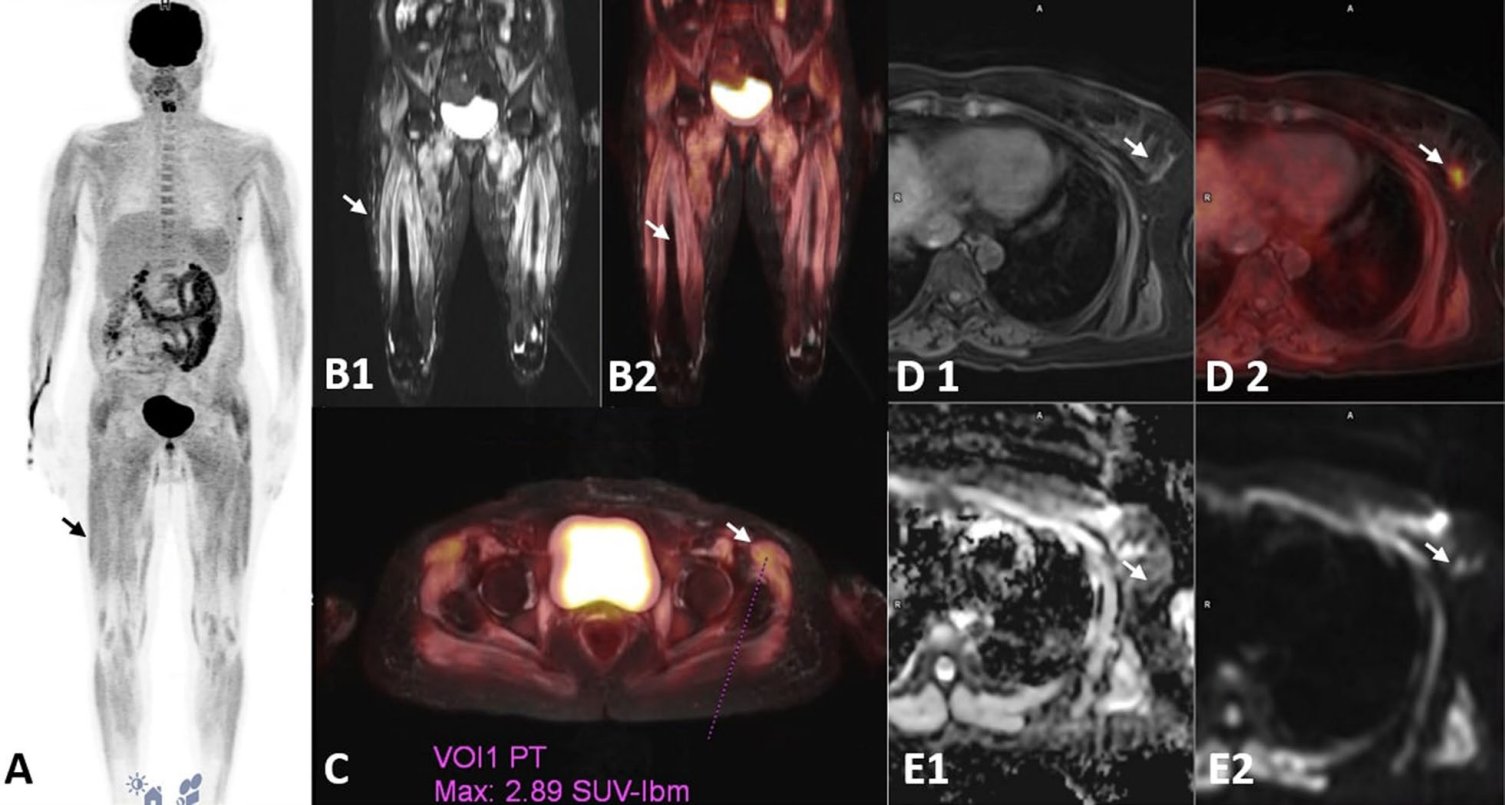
PET-MRI images of a 48-year-old lady with Dermatomyositis and Carcinoma Breast. A Whole body MIP image (black arrow). B1 Coronal T2 FS BLADE MR image showing hyperintensity changes in the proximal thigh muscles (white arrow). B2 Coronal fused PET/MRI image shows increased FDG uptake in all the proximal thigh muscles (white arrow). C Axial Fused T2FS BLADE MR/PET images showing increased FDG uptake in the hip girdle muscles with SUV max of 2.89 (white arrow). D1 and D2. Axial Fused T1 VIBE DIXON (W) and fused PET/MR images showing focal lesion with increased FDG uptake in lower outer quadrant of the left breast (white arrow). E1 and E2. Axial ADC and TRACE DWI MR images showing focal area of diffusion restriction corresponding to the focal lesion on PET (white arrow)
